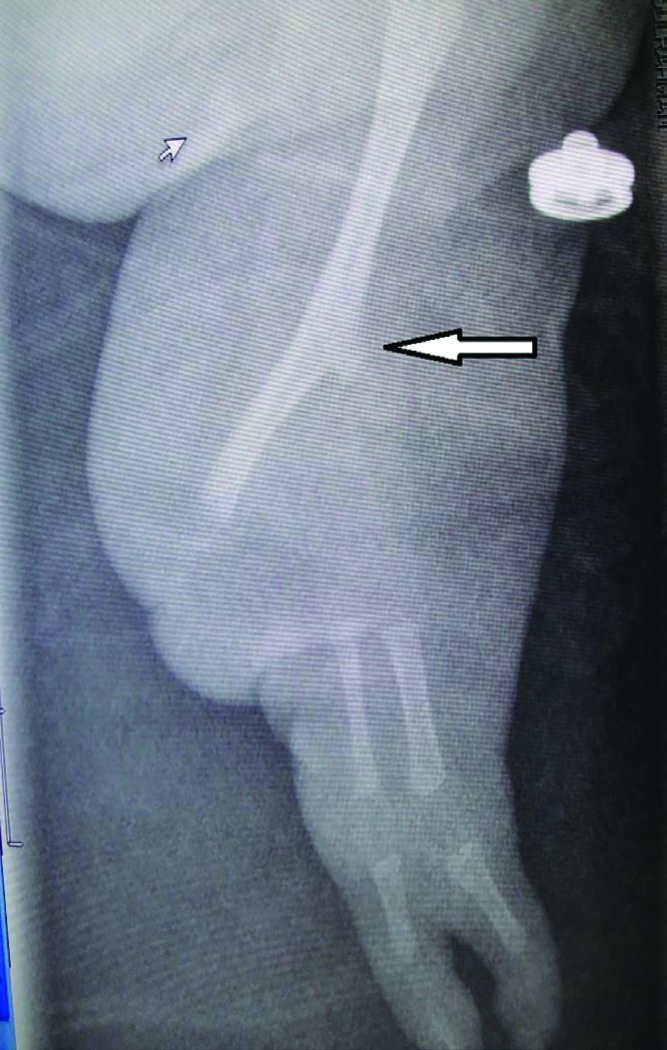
X-ray of the left upper limb showing ulnar ray deficiency. Note the radio-humeral synostosis (the area of synostosis has a prominent humeral medial epicondyle and is marked with an arrow), the absent ulna, and the two digital rays in the hand
